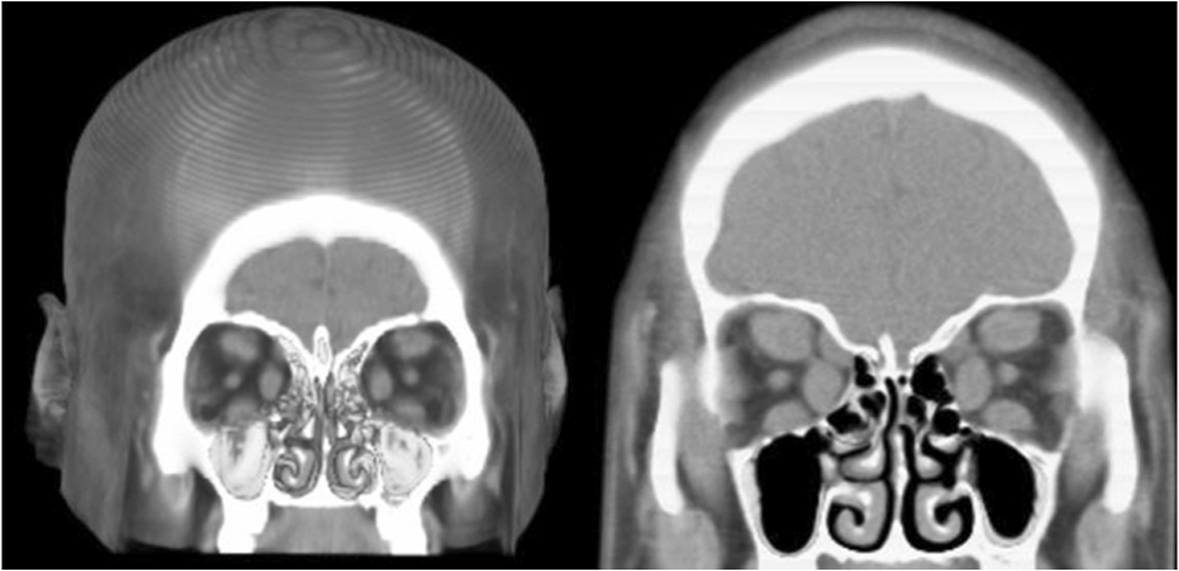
The thickening of extraocular muscles was evaluated on multislice CT with volumetric reconstruction of a coronal slice. Left: thickening is evident in the inferior, medial, and superior rectus muscles. Right: thickening of the four rectus muscles and SO.
